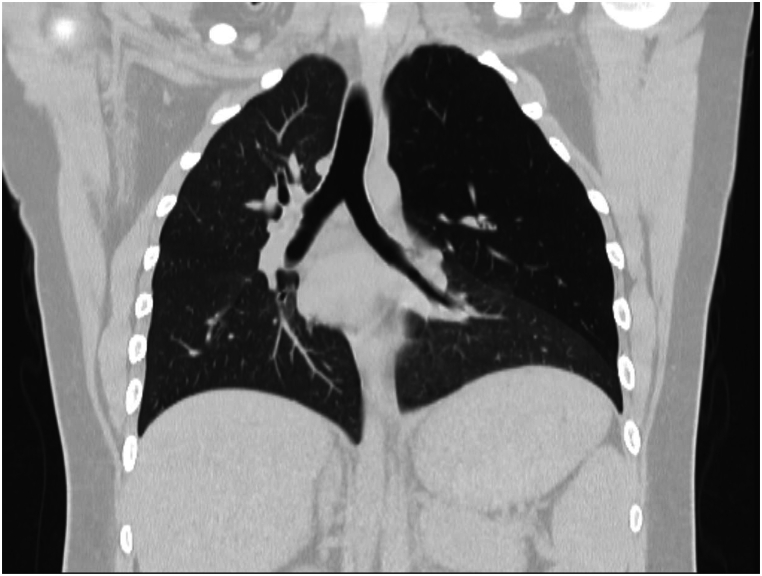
Coronal HRCT. Entire part of left upper lobe including superior and inferior segments of lingula demonstrates hyperinflation associated with diminished lung markings. Pressure effect over mediastinal structures is seen secondary to ball-valve mechanism.
